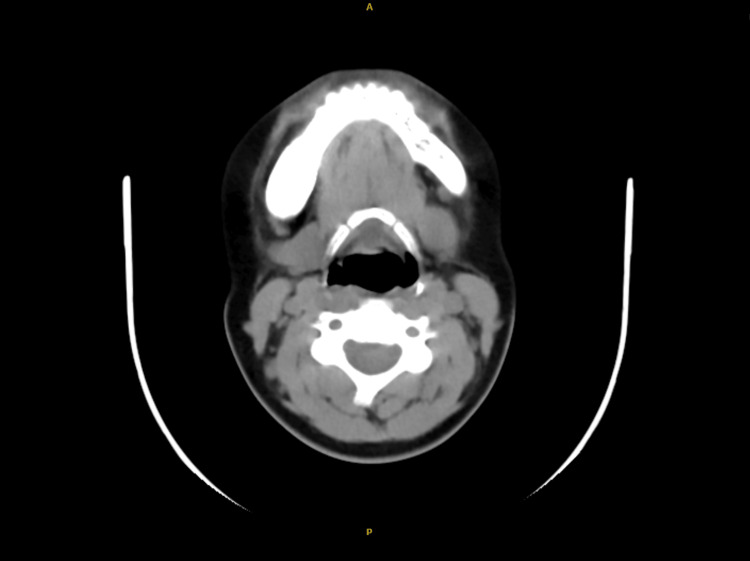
Plain neck computed tomography (CT) scan Fibrous dysplasia of the right mandible with involvement of the right alveolar foramen and oedematous changes of the right masseter muscle, sparring the right temporomandibular joint.
